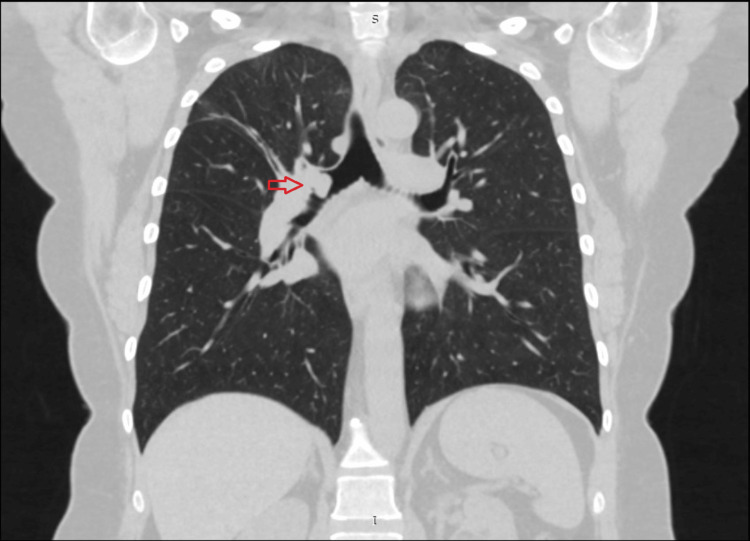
Diagnostic CT scan showing intraluminal mass (arrow)
